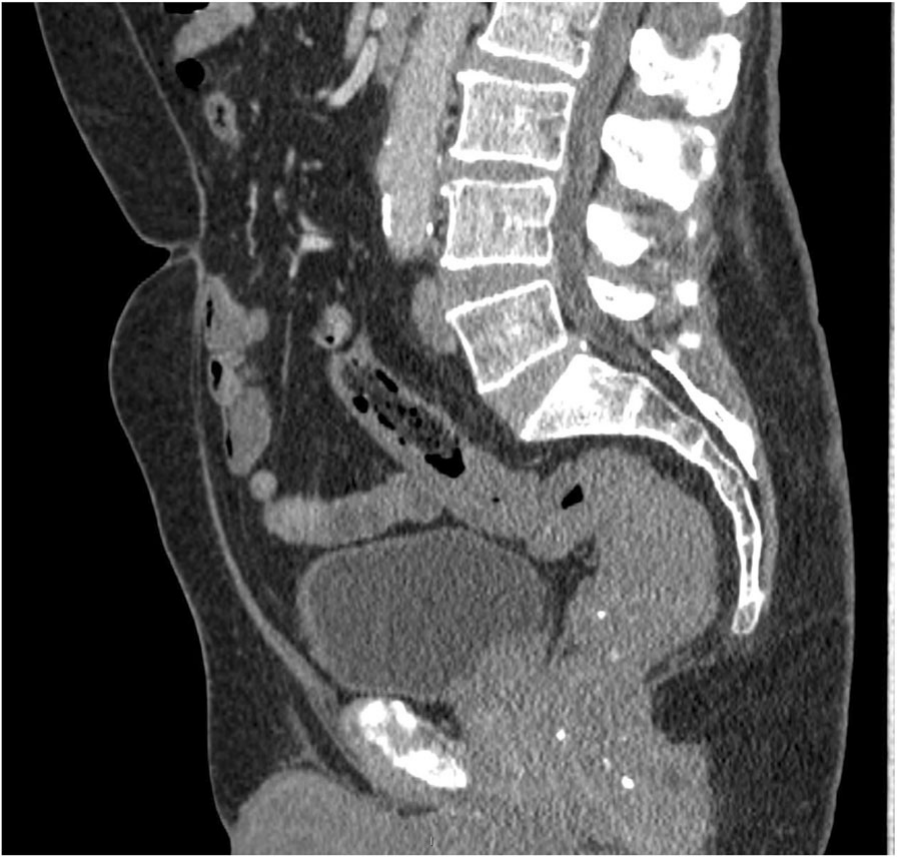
Sagittal contrast-enhanced CT abdomen and pelvis. Irregular and eccentric thickening of the rectum. Multiple calcified phleboliths. Relatively abrupt transition to normal rectal wall at the rectosigmoid junction
